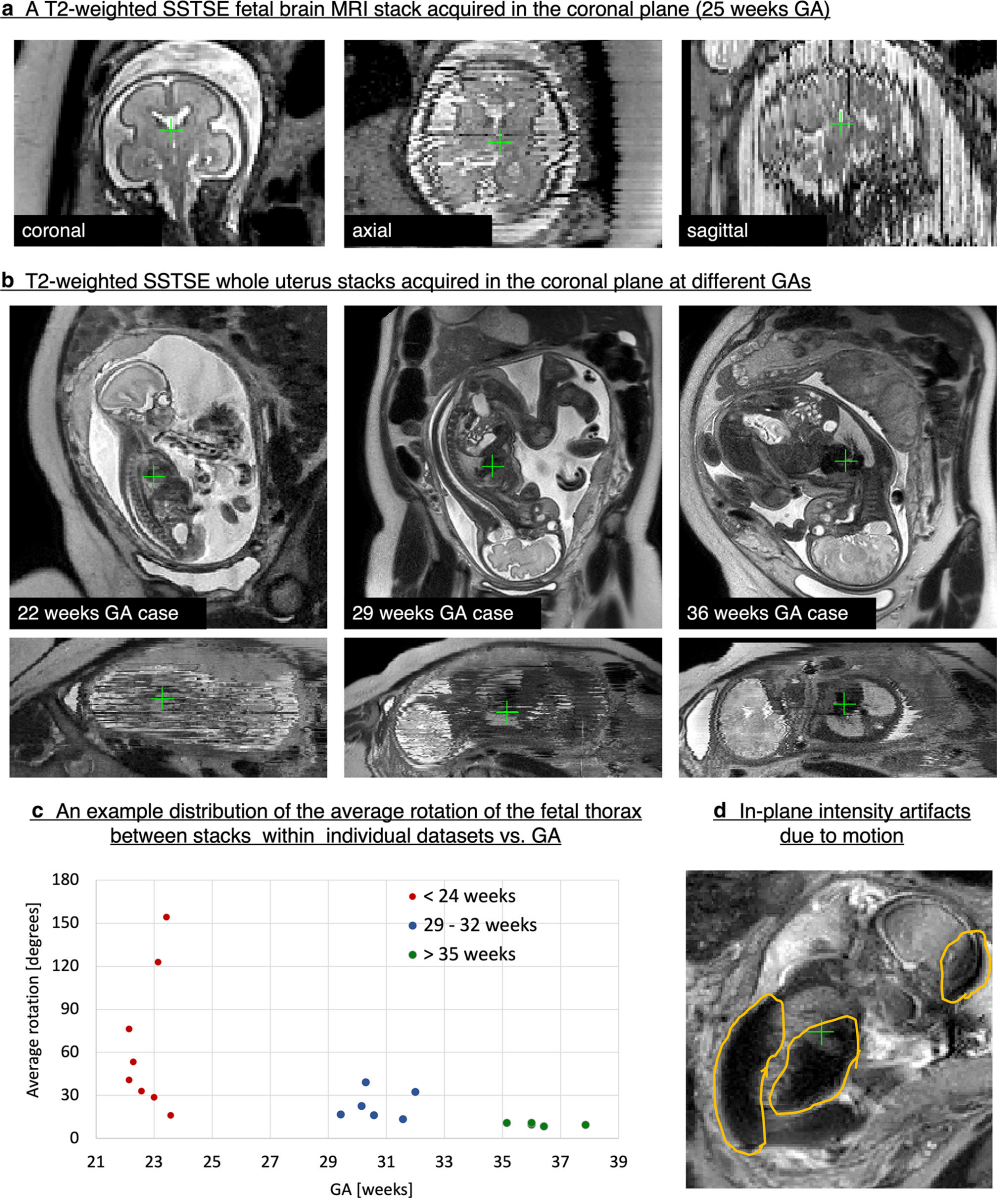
Motion in foetal MRI: examples of *T2*-weighted SSTSE stacks of 2D slices. The MRI datasets used in this example were acquired at St.Thomas’ Hospital, London.
